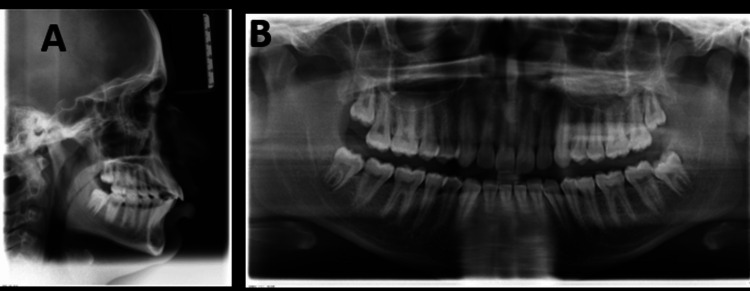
Pretreatment (A) cephalometric radiograph and (B) orthopantomogram
